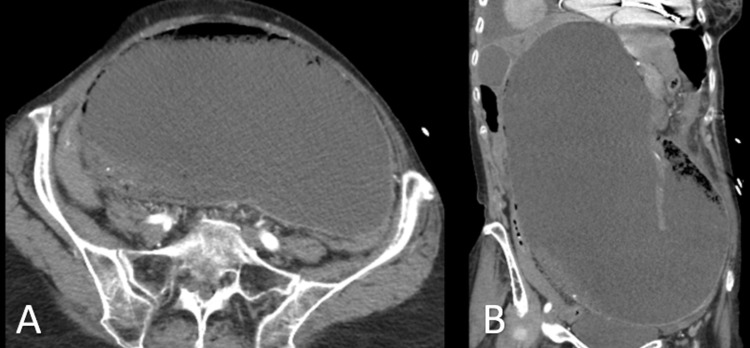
CT of the abdomen without contrast Imaging demonstrates markedly dilated stomach in (A) transverse view and in (B) coronal view.
